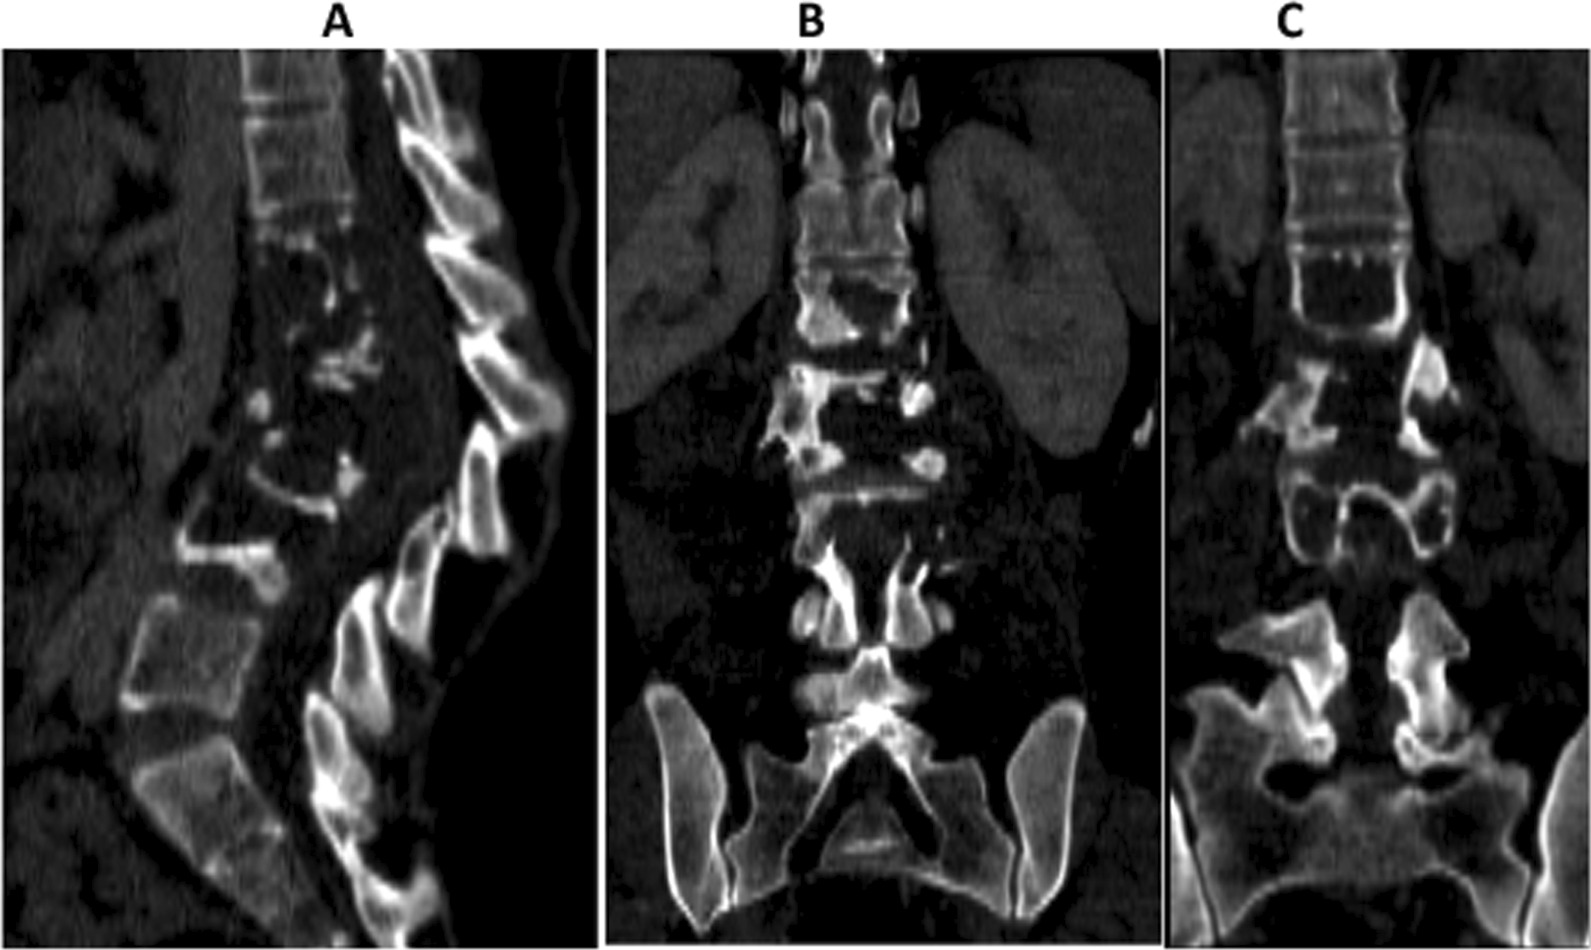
Abdominopelvic CT scan bone window: sagittal (A) and coronal (B & C) plane images show extensive lytic destruction of the L1–L4 vertebral bodies, loss of corresponding intervertebral disc spaces and kyphotic deformity of the affected lumbar spine
